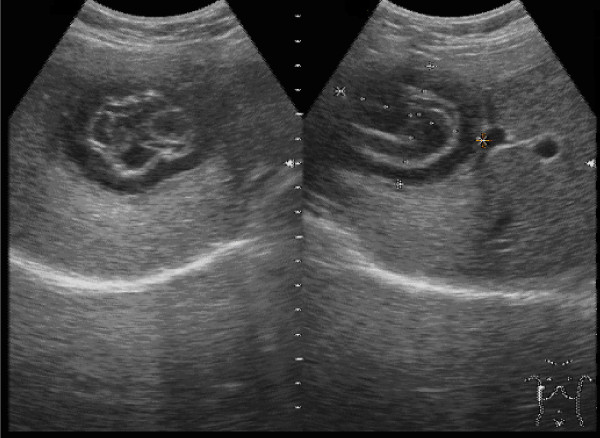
Sonographic appearance of the echinococcal cyst in hepatic segment VIII.
